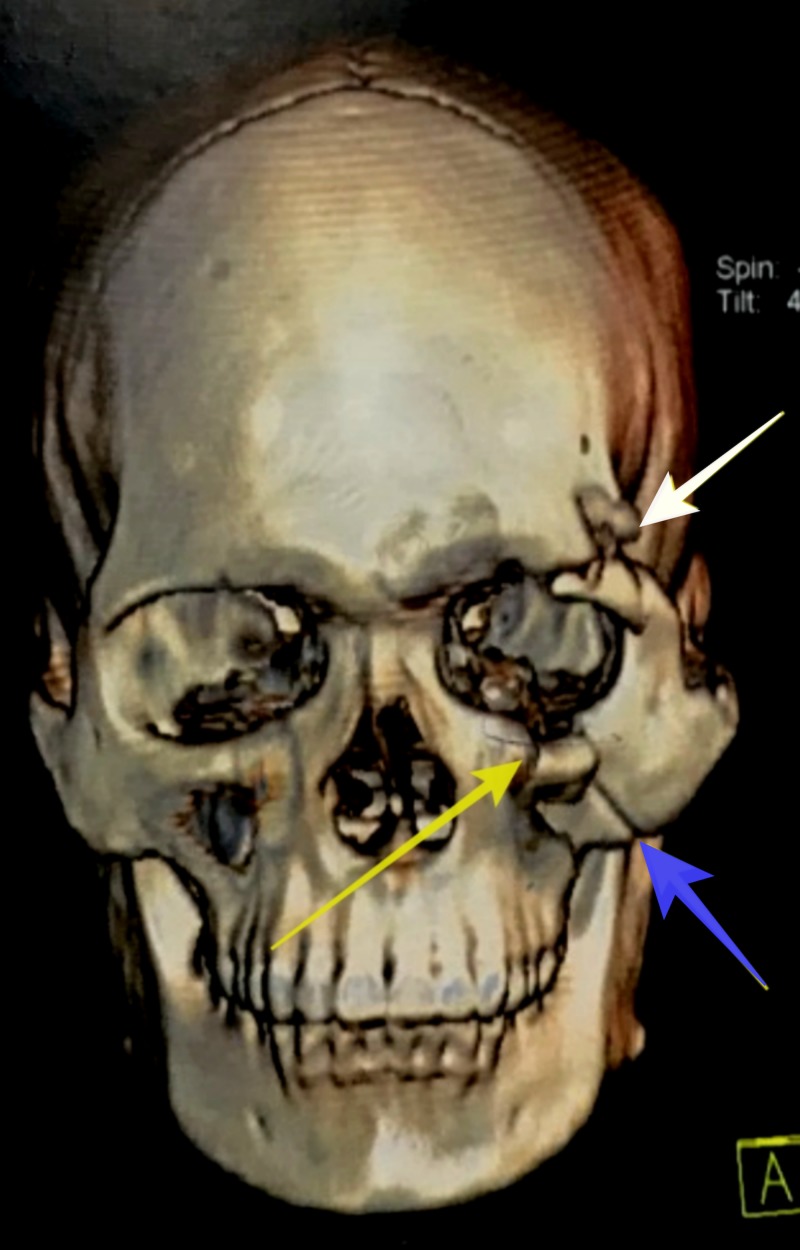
Virtual three-dimensional reconstruction of computed tomography scan demonstrating fracture at left front zygomatic region (white arrow), left zygomatic maxillary buttress (blue arrow), left infra orbital rim (yellow arrow).
